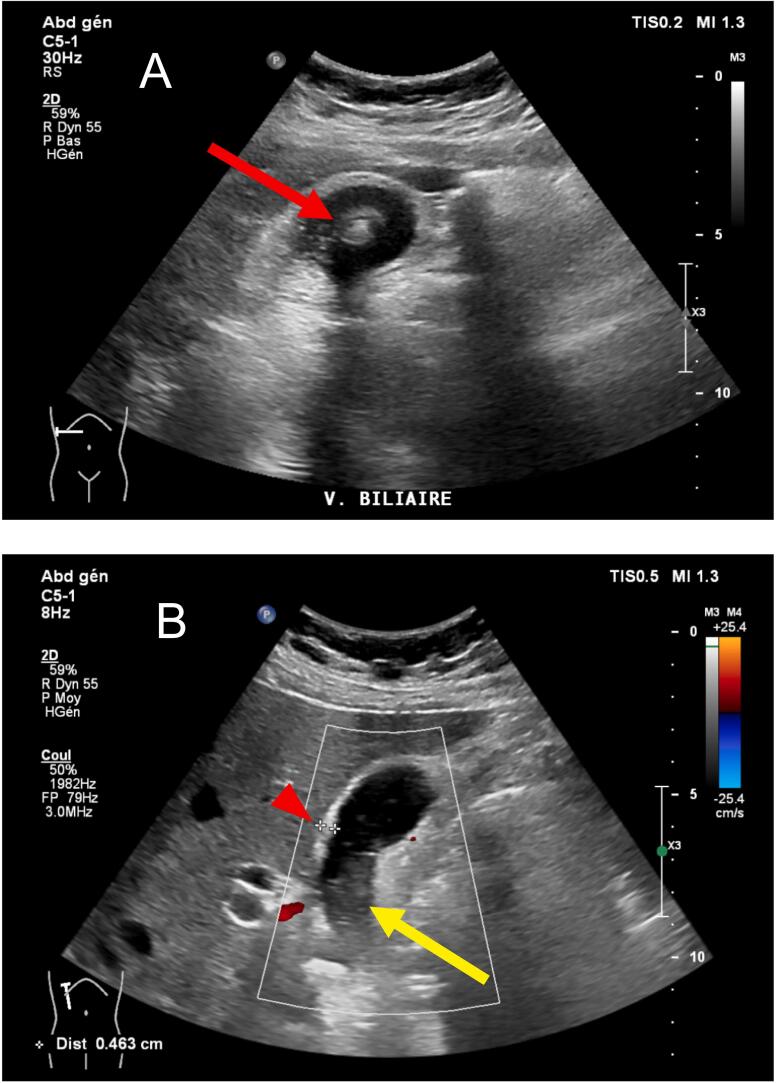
Preoperative sonography. (A) Lithiasic cholecystitis with a stone of 26 mm (red arrow). (B) Sludge (yellow arrow), thickening of the gallbladder of 4.6 mm (red arrowhead).
